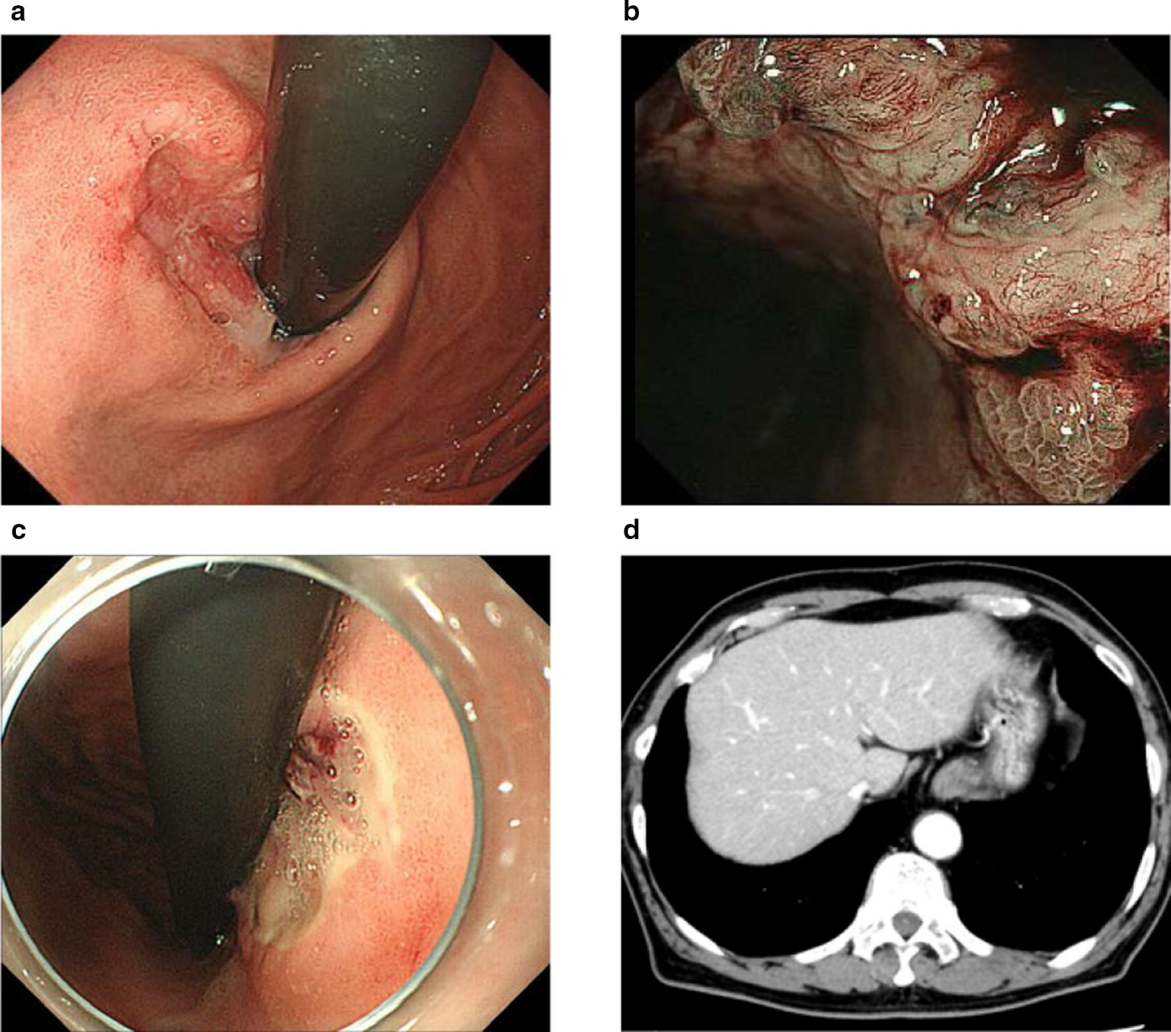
a Esophagogastroduodenoscopy shows an ulcerated tumor at the gastric cardia. b Narrow-band imaging of tumor shows irregular microvascular and microsurface patterns with wavy micro-vessels. c Esophagogastroduodenoscopy also shows an ulcer with exposed vessel at the mid-body of the stomach. d Computed tomography of the abdomen and pelvis shows no thickening of the stomach wall and no lymph node enlargement
